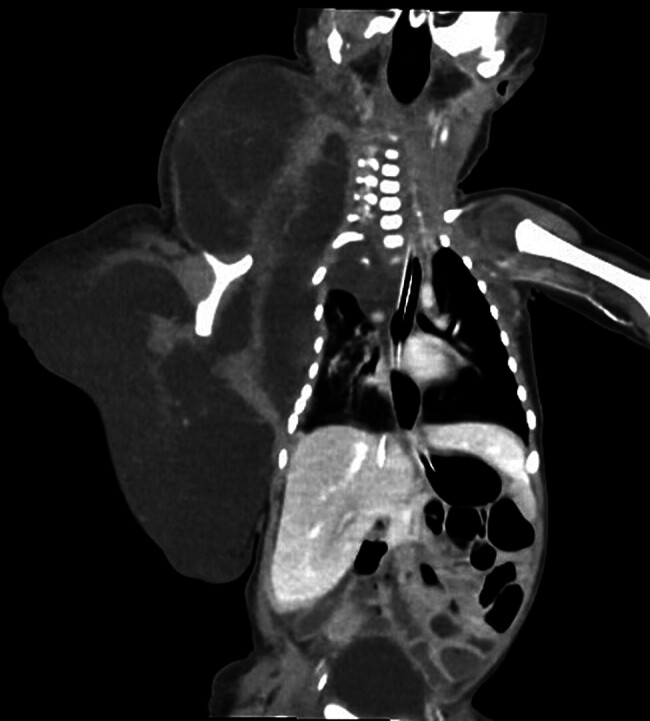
Chest computed tomography (CT) showing a large multiloculated cystic lesion in the right cervicothoracic region, displacing adjacent structures and compressing the sternocleidomastoid muscle, parotid, and submandibular glands. Subsegmental atelectasis is observed in the lower right lung lobe, with no evidence of bone involvement. Findings are consistent with a giant cystic lymphangioma.
